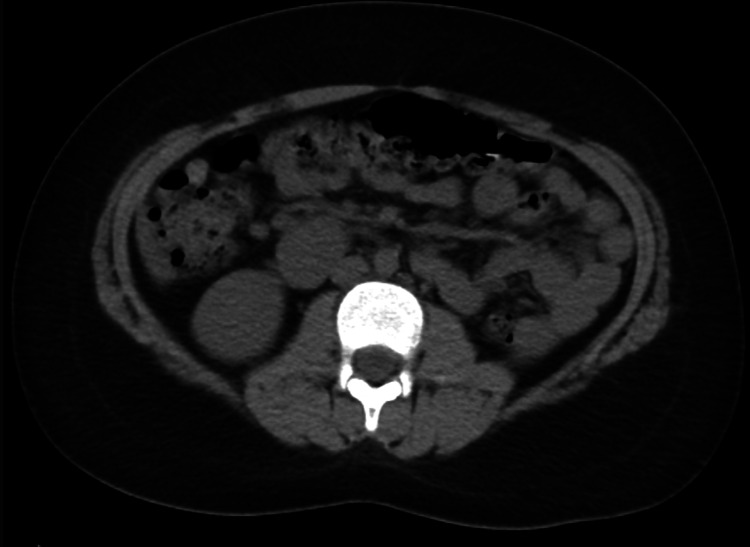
Axial CT image demonstrates crossed left renal ectopia
